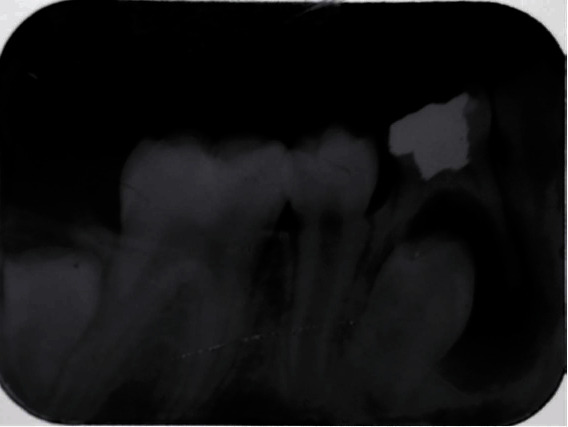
Retroalveolar radiograph showing radiolucency associated with an endodontically treated first right primary molar (tooth 84).
